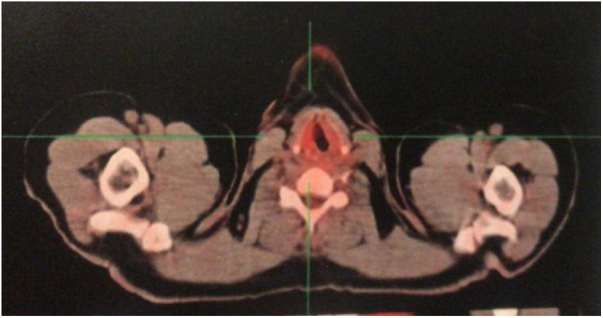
PET-CT, axial section of the cervical region. Discrete asymmetric radioconcentration.
